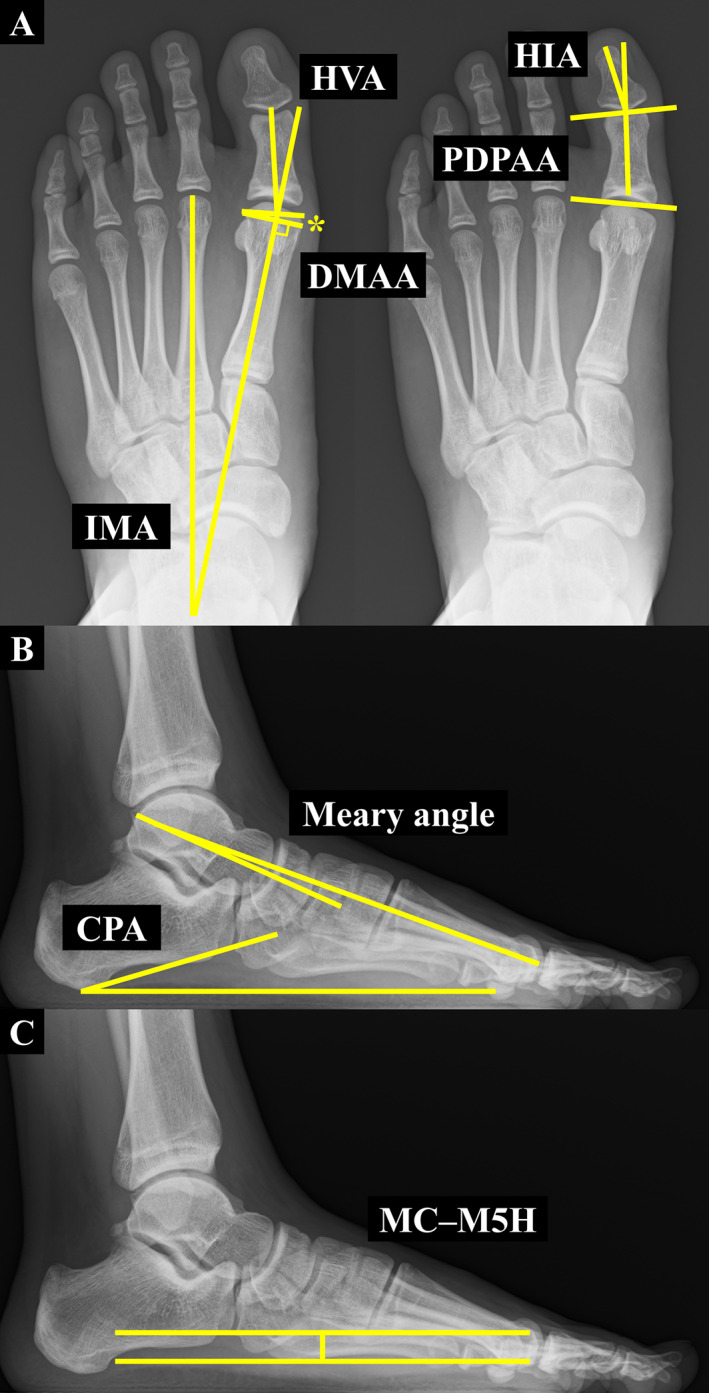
Representative radiographic measurements. (A) Weight‐bearing dorsoplantar radiographs demonstrating measurement of the hallux valgus angle (HVA), intermetatarsal angle (IMA), distal metatarsal articular angle (DMAA), hallux interphalangeal angle (HIA), and proximal–distal phalanx articular angle (PDPAA). PDPAA was defined as the angle between the lines representing the proximal and distal articular surfaces of the proximal phalanx. (B) Weight‐bearing lateral radiograph demonstrating measurement of Meary angle and calcaneal pitch angle (CPA). (C) Medial cuneiform–fifth metatarsal height (MC–M5H).
